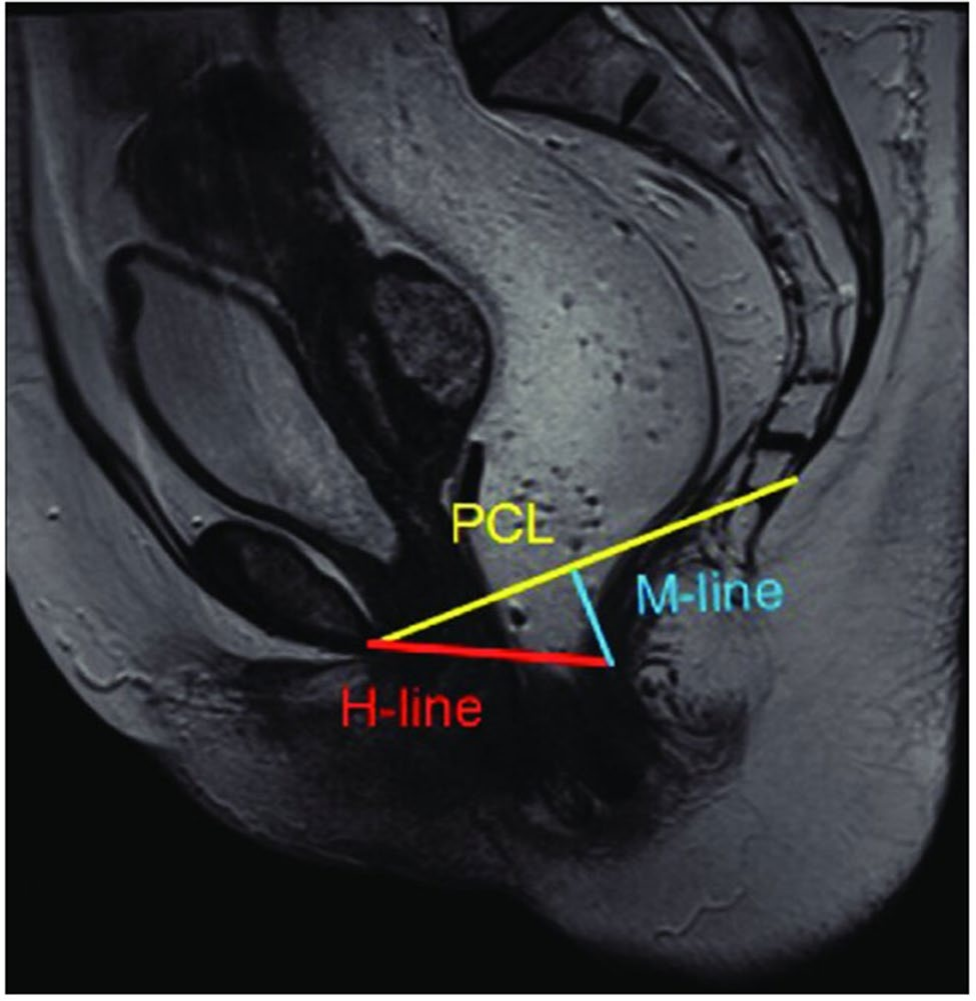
T2W sagittal section of the pelvic magnetic resonance imaging depicting the normal pubococcygeal line (PCL) (yellow), H line (red) and the M line (blue). PCL is between the inferior margin of symphysis pubis and the tip of the coccyx. H-line is the distance between the inferior border of the pubic symphysis and the posterior wall of the rectum at the level of the anorectal junction and indicates the width of the levator hiatus. M-line is the vertical line drawn perpendicularly from the PCL to the posterior end of the H line. M line indicates the degree of decent of the levator hiatus or the degree of pelvic floor laxity
